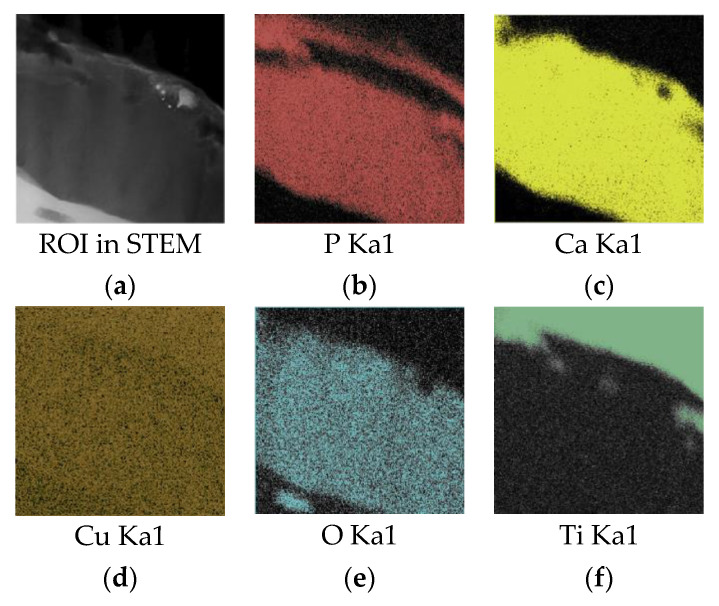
An energy dispersive X-ray (EDX) element mapping of the Cu-HA and Ti substrate in TEM lamella. (a) ROI in STEM; (b) P Ka1; (c) Ca Ka1; (d) Cu Ka1; (e) O Ka1; (f) Ti Ka1.
